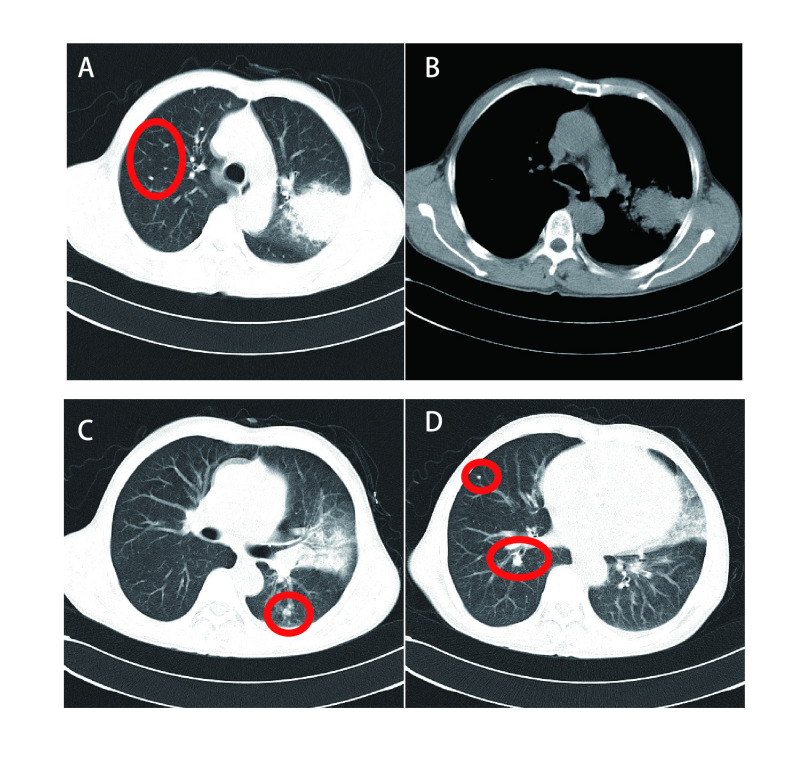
胸部CT：双肺多发病变、边缘不清。A、B、C、D为同一患者：左肺上叶后段、舌叶见大片密度增高影，边界模糊，密度不均，内有小片低密度影，余双肺见多发斑点状密度增高影（红色圈所圈部位），左侧少量胸腔积液。 Chest CT: multiple lesions and unclear edges. A, B, C, D are the same patient: A large high density flake shadow with small pieces of low density shadow is showed in the rear section and tongue of left lung upper, boundary blur, uneven density, the remaining double lungs see multiple spots high density shadow (red circle area), a small amount of chest fluid on the left.
